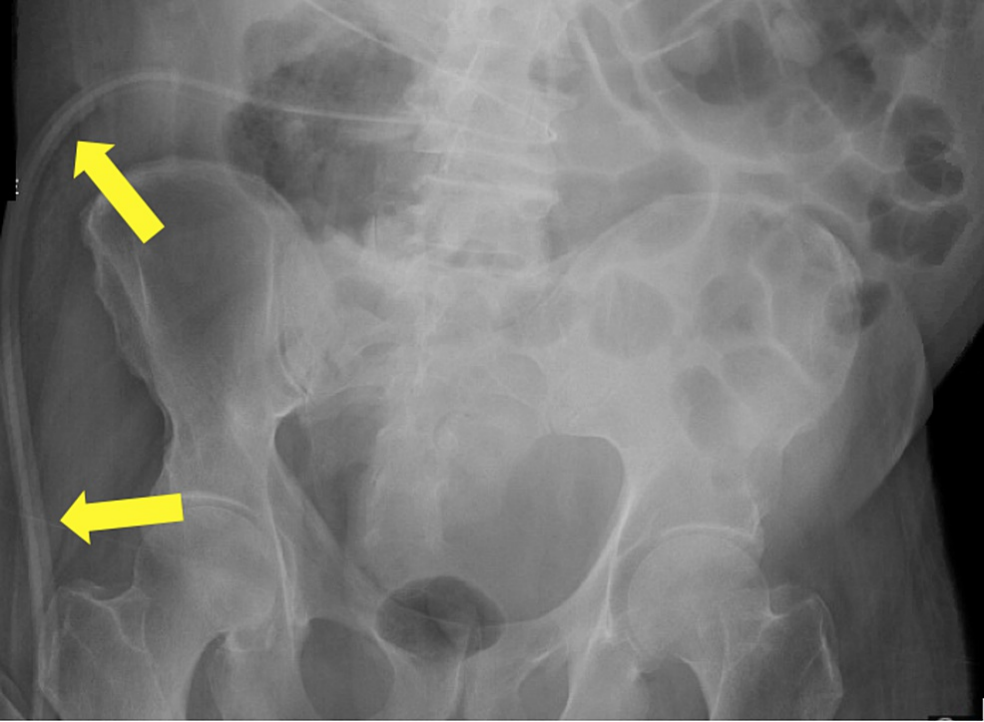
Abdominal x-ray (AP supine view) demonstrates a Jackson Pratt (JP) drain after open cholecystectomy. Note the drain (yellow arrows). AP - anteroposterior
